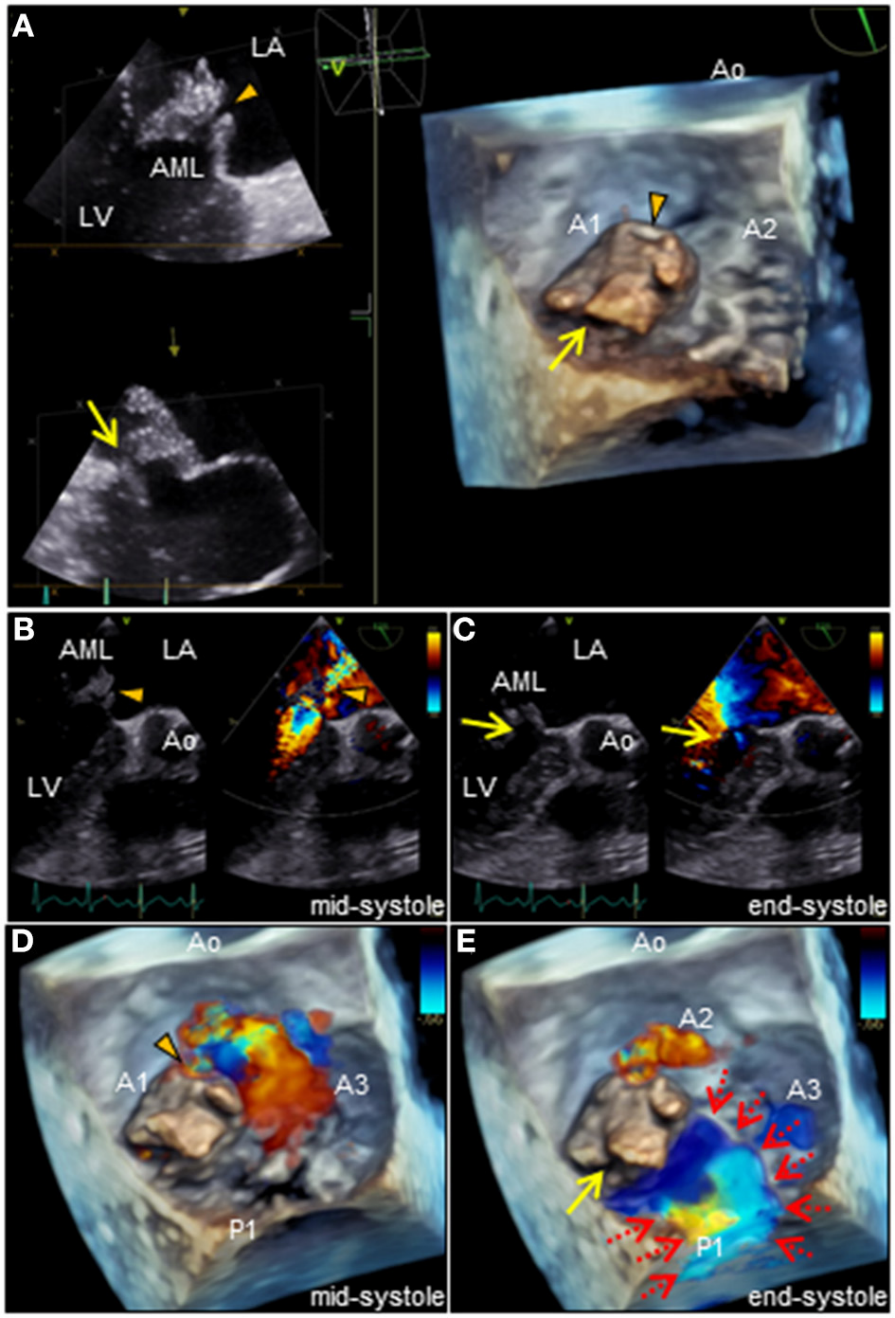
(A) Three-dimensional TEE of the mitral valve. Note the two distinct ruptured perforations through the MVA (arrowhead and arrow, respectively). (B–E) Two or three-dimensional color Doppler TEE reveals that severe MR with two different jets communicate with the LA through the MVA: a superior jet (arrowhead) and a posterior jet (arrow), respectively. Note the MR with posterior jet heading toward the LA via the PML surface (dotted arrows). AML, anterior mitral leaflet; Ao, aorta; LA, left atrium; LV, left ventricle; MR, mitral regurgitation; PML, posterior mitral leaflet; MVA, mitral valve aneurysm, TEE, transesophageal echocardiography.
